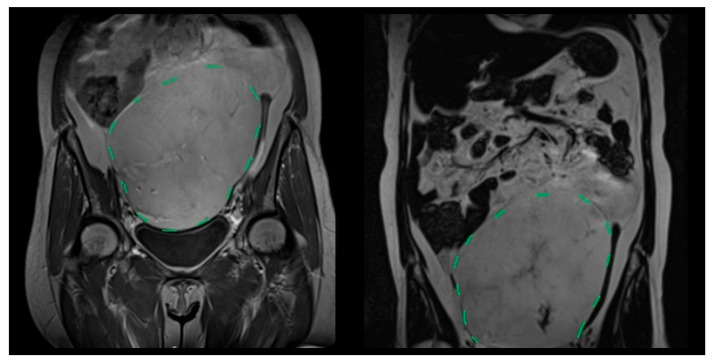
Coronal T2-weighted MRI showing a giant primary mesenteric liposarcoma extending from the pelvis to the upper abdomen (green dashed line indicates the tumor boundary).
